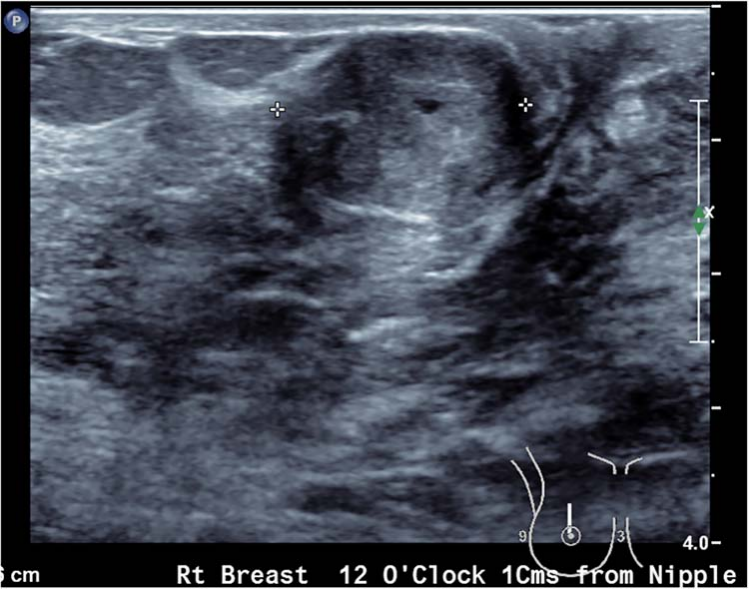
Ultrasound image of the ovoid breast lesion.
